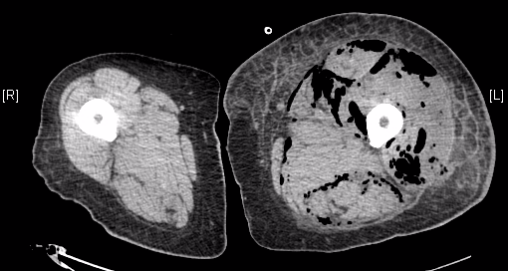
Computed tomography demonstrating gas in all muscle compartments of the left thigh.
